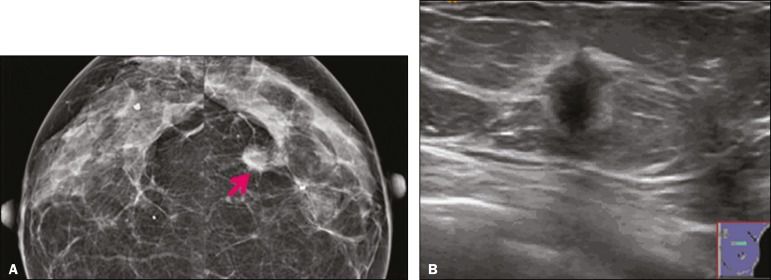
Digital mammography, in a craniocaudal view (A), ultrasound (B) in a 56-year-old woman (case 5) with GCT of the breast. Note the heterogeneous nodular density projecting into the transition between the upper quadrants of the left breast, with mixed density and some fine spiculated margins. In this heterogeneously dense parenchyma, the mass became visible because it protruded into the more adipose portion of the breast tissue. The mass was growing deep into the tissue and presented as an hypoechoic nodule (B) with an irregular shape and spiculated margins. It is surrounded by an hyperechoic halo, as described by Yang et al.^((20))^ as a possible consistent, typical finding of GTC of the breast.
